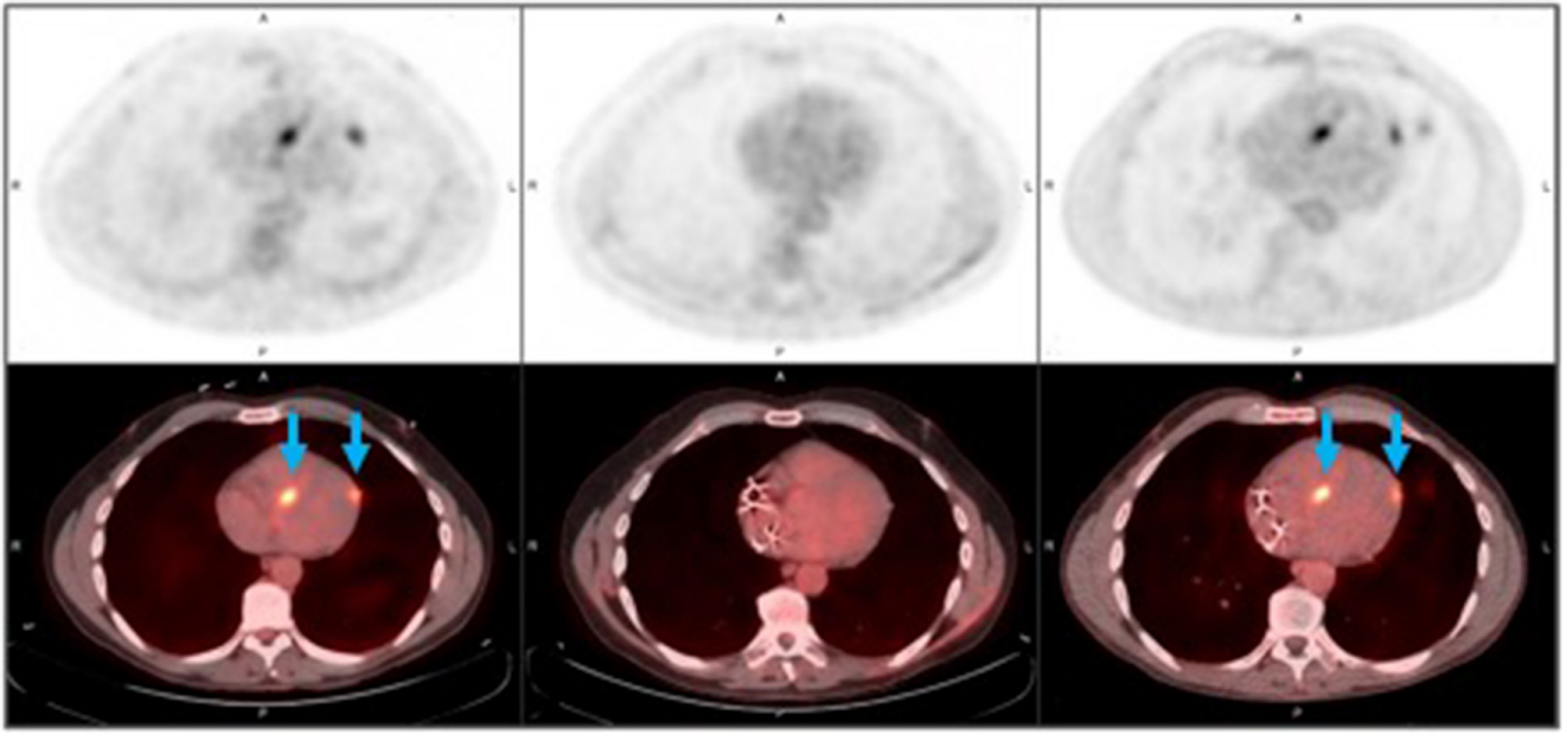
Recurrent cardiac sarcoidosis. These [^18^F]FDG-PET/CT axial images observed in a 53 year-old male represent findings in recurrent cardiac sarcoidosis. The top row demonstrates FDG-PET images, and the bottom row demonstrates FDG-PET images fused with CT imaging. Foci of increased FDG uptake (blue arrows) are representative findings in recurrent cardiac sarcoidosis present in the mid septal and mid lateral walls (first column), then resolve with therapy (middle column), and then recur in the same location (third column). By column, images are displayed chronologically. The left column captures pre-treatment disease features; these can be compared to imaging features of disease recurrence depicted in the right column (performed 10 months after the initial, pre-treatment scan). Images in the middle column negative scan (performed 5 months after the initial, pre-treatment scan) demonstrate the disappearance of metabolically-manifest disease activity.
